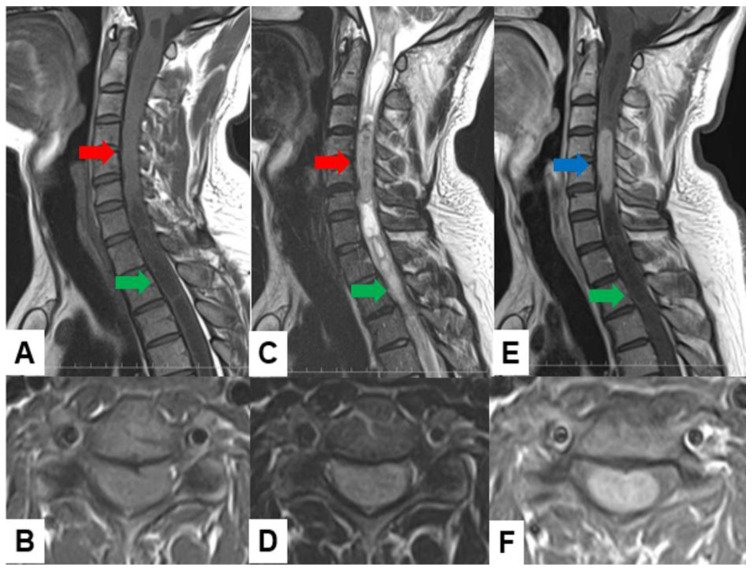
Thirty-seven M, spinal ependymoma, grade 1. (A) T1-weighted midsagittal image, (B) T1-weighted axial image at C4/5, (C) T2-weighted midsagittal image, (D) T2-weighted axial image at C4/5, (E) enhanced T1-weighted midsagittal image, and (F) enhanced T1-weighted axial image at C4/5. Red arrows indicate tumor; blue arrow shows enhancement. Green arrows indicate large syringomyelia.
